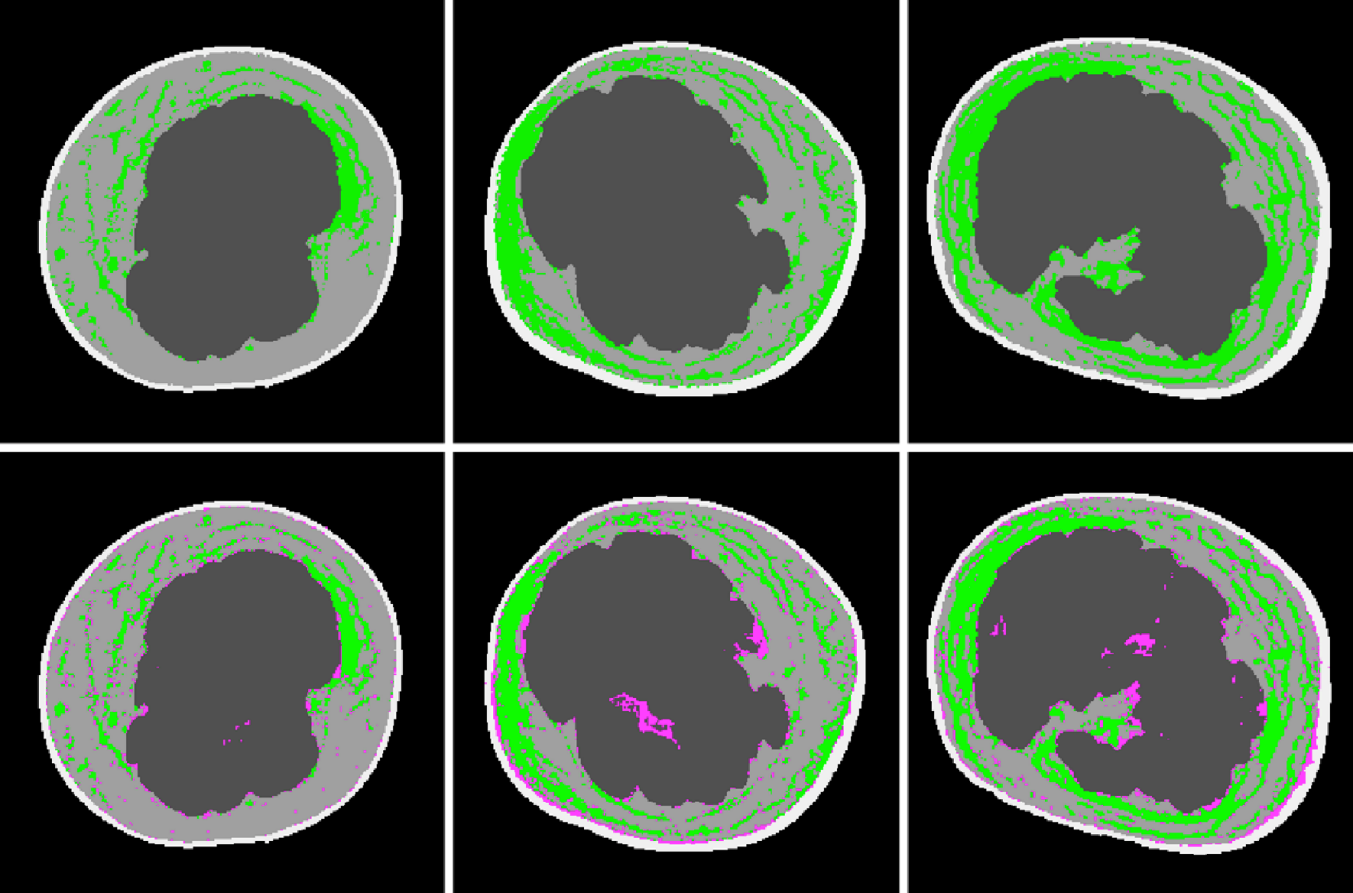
CT images (upper row) labeled using the lab-developed GUI and images classified using the trained algorithm (lower row). The pixels classified as fibrosis are marked in green, and the pixels incorrectly classified as fibrosis are marked in red.
